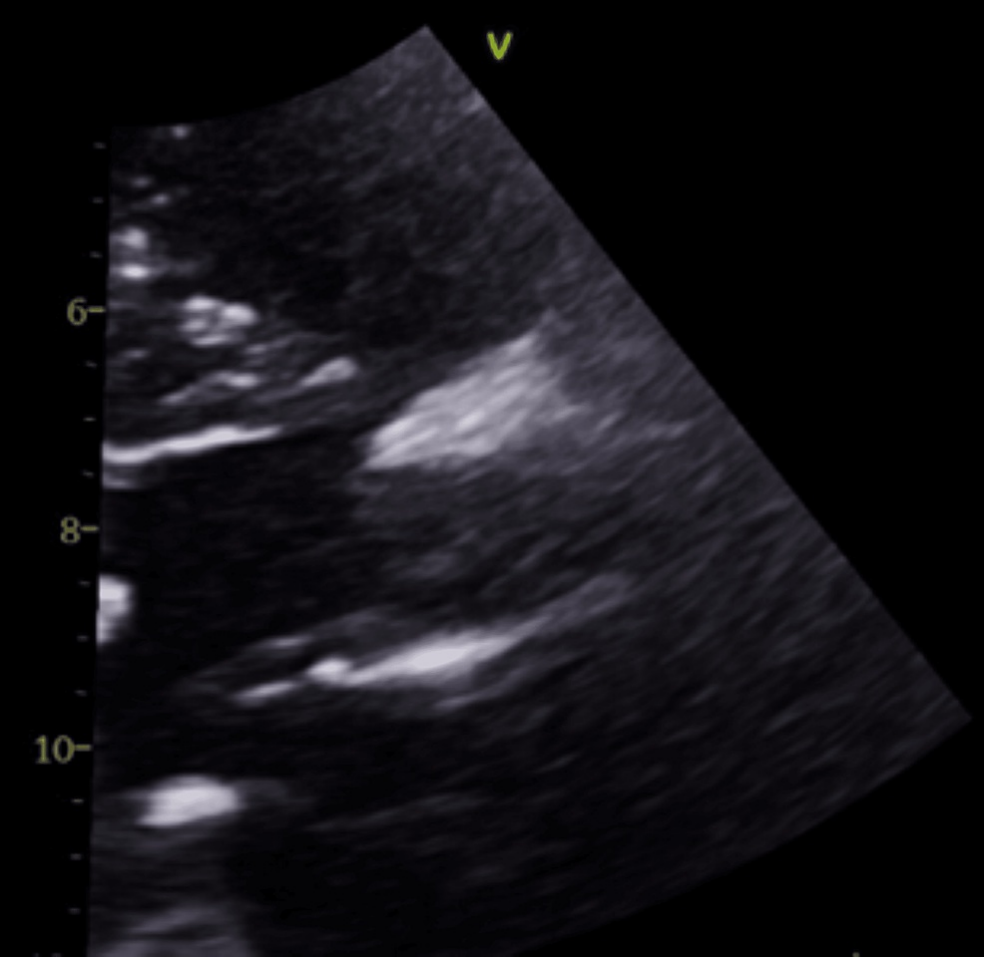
Post-TAVR Parasternal Long-Axis View Image taken one day following procedure. TAVR: transcatheter aortic valve replacement
